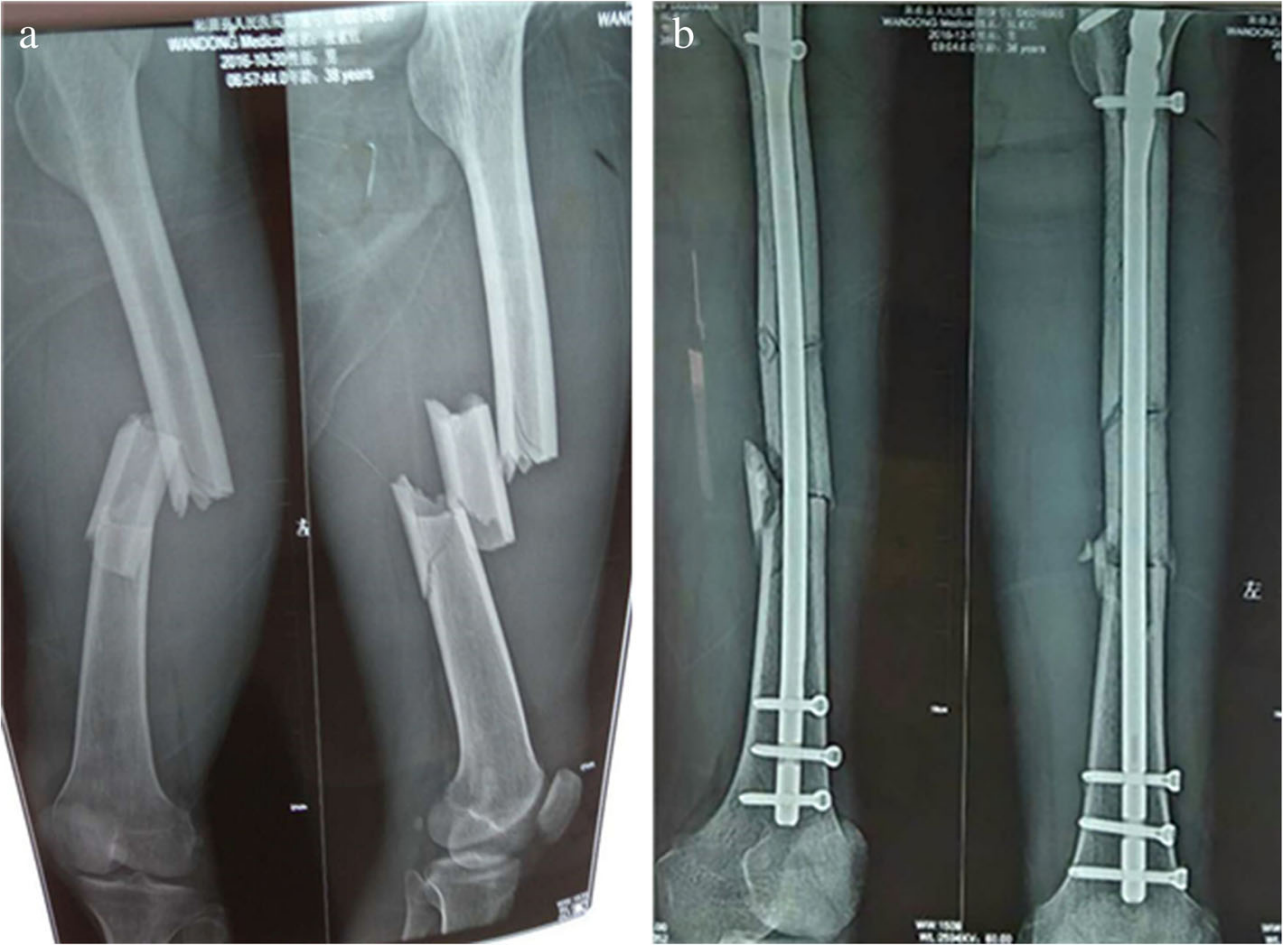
Preoperative and postoperative images a Multi-segment fracture of the femoral shaft: preoperative X-ray film. b Postoperative X-rays after the application of the external frame-assisted reduction
